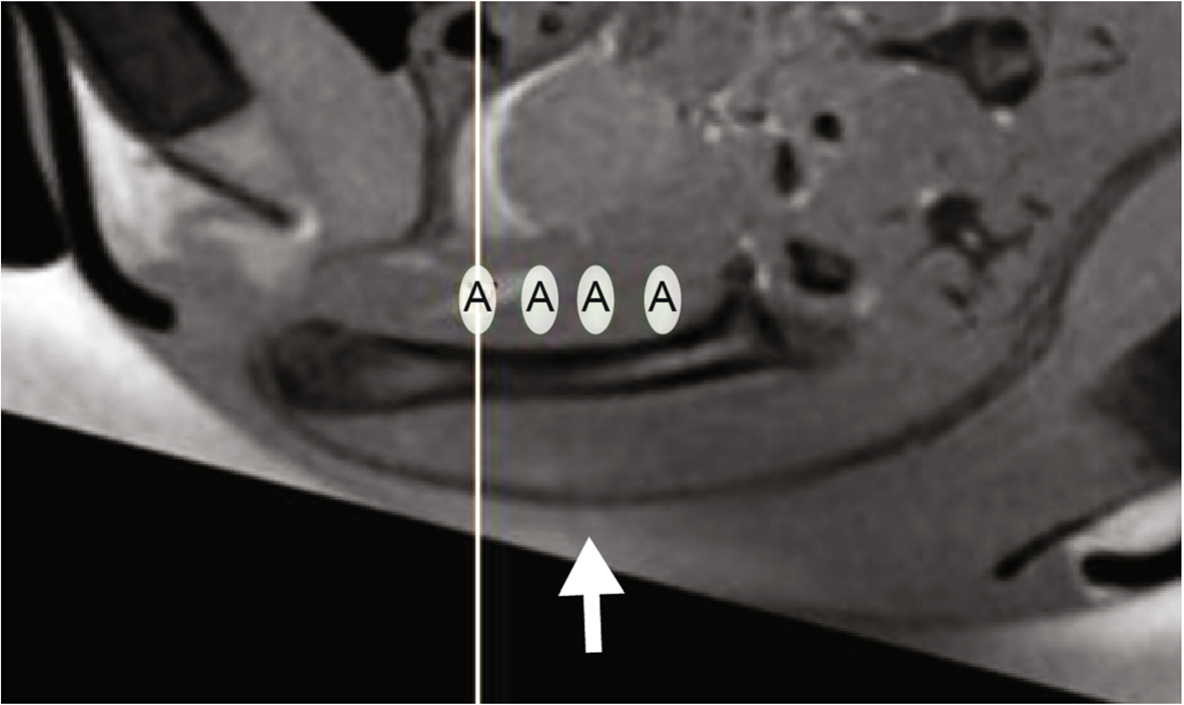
Representative T_1_-weighted image for position of treatment cells for MR-HIFU ablation. Direction of ultrasound beam (*white arrow*). Treatment cells (*A*)
